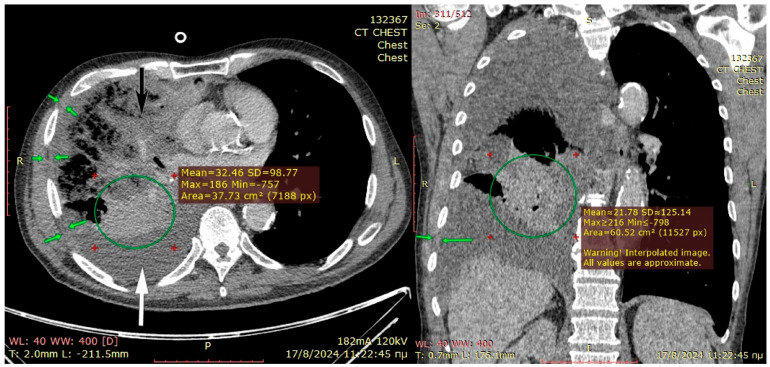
Preoperative planning CT-scan for Case 2 where the severely infiltrated middle lobe (black arrow) mandates salvage lobectomy to avoid gangrenous conversion and sepsis. The lower right lobe (green circle) is grossly atelectatic, but assessing its viability and attempting decortication is only possible through thoracotomy. The green arrows in both pictures show a moderate chest wall thickness. The decision for Sawamura can only be undertaken intraoperatively. An open thoracostomy window could also be offered to this patient if only the community can offer daily dressing changes.
